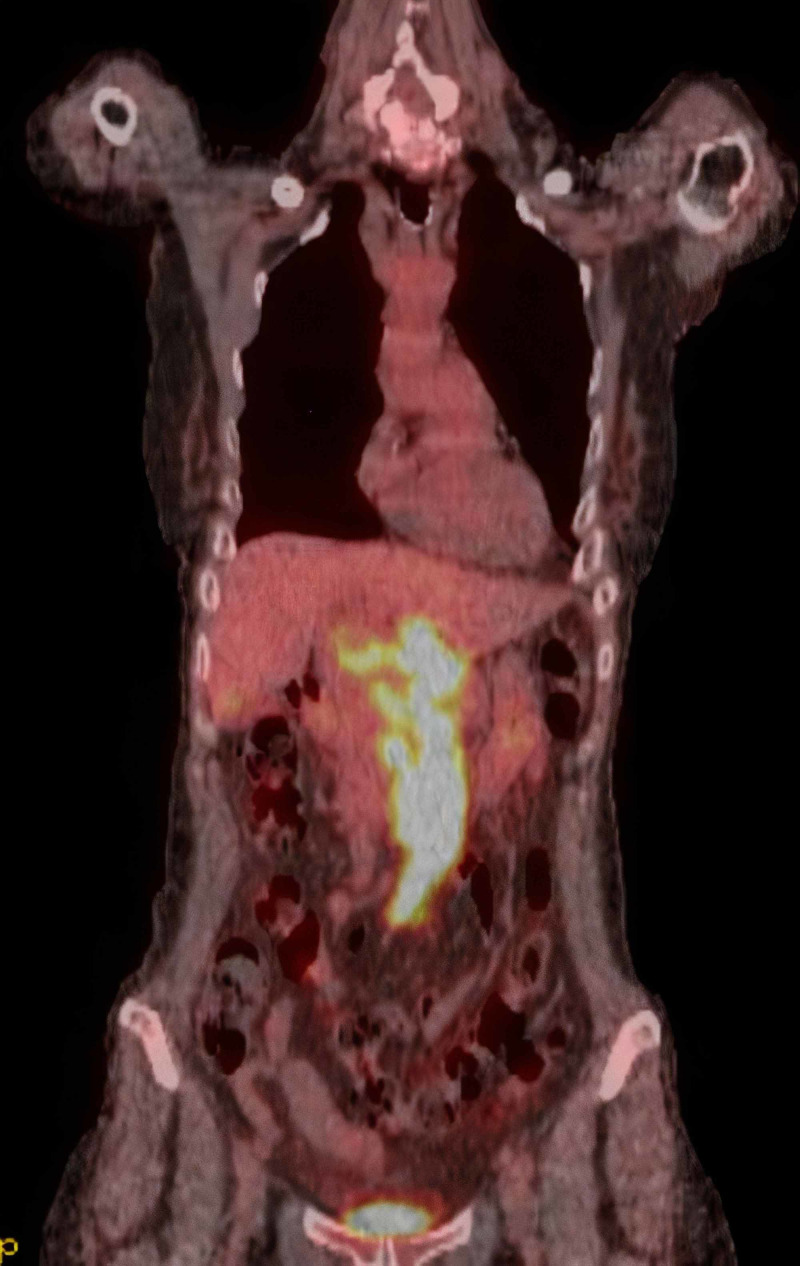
Positron emission tomography/CT scan showing a localized 18 F-fluorodeoxyglucose (FDG)-avid mass surrounding the portal vein.
